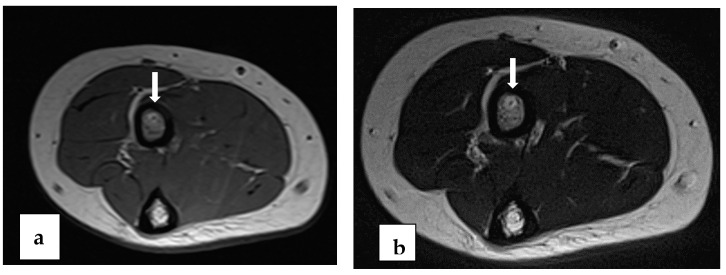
T1-weighted (a) and T2-weighted (b) axial images with bright halo (white arrow) surrounding a faint residual of BIN in radial bone.
